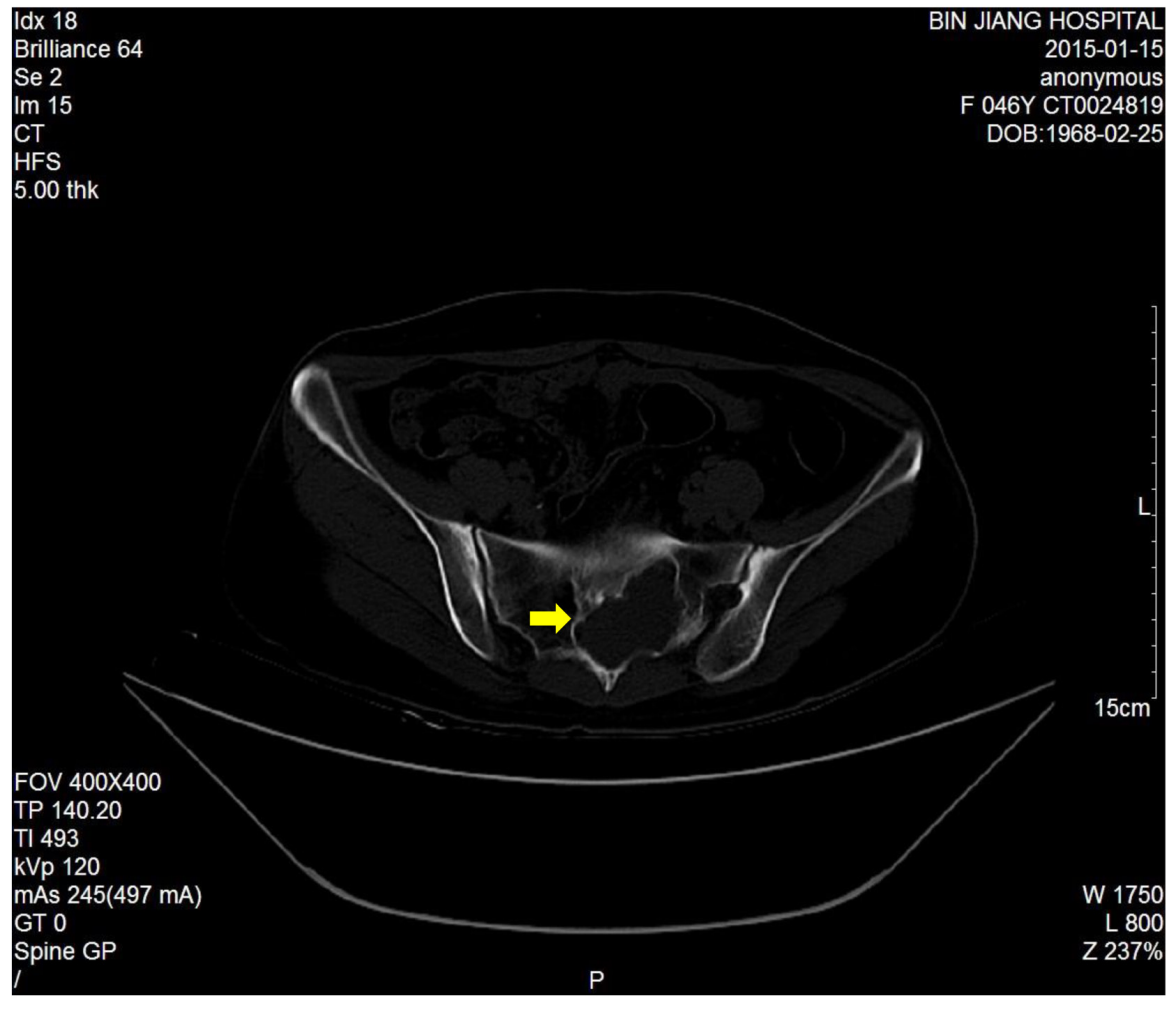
Axial CT scan showed a huge left sacral expansive lesions with marginal sclerosis(S1-2)
